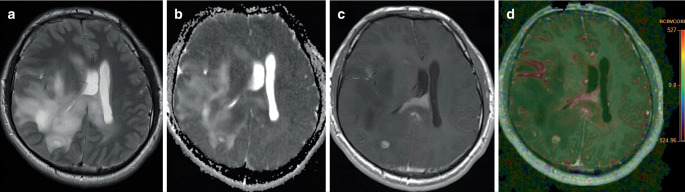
a–d Diffuse astrocytoma with mutation of isocitrate dehydrogenase (IDH-mutation) and cyclin dependent kinase 2A homozygous deletion (CDKN2A homozygous deletion), World Health Organization grade 4 (WHO grade 4) (male, age: 24 years) showing a growth pattern of gliomatosis cerebri with involvement of large parts of the right hemisphere with midline deviation (a), areas with low values for the apparent diffusion coefficient (ADC values) indicating high cellularity (b). Only focal contrast enhancement (c), corresponding high values for the relative cerebral blood volume (rCBV values) (d). a T2-weighted image, b ADC map, c T1-weighted image after i.v. application of contrast agent, colored rCBV map, respectively. Presence of a homozygous deletion of CDKN2A/B is a criterion for grading a diffusely infiltrating IDH-mutant astrocytoma as WHO tumor grade 4
